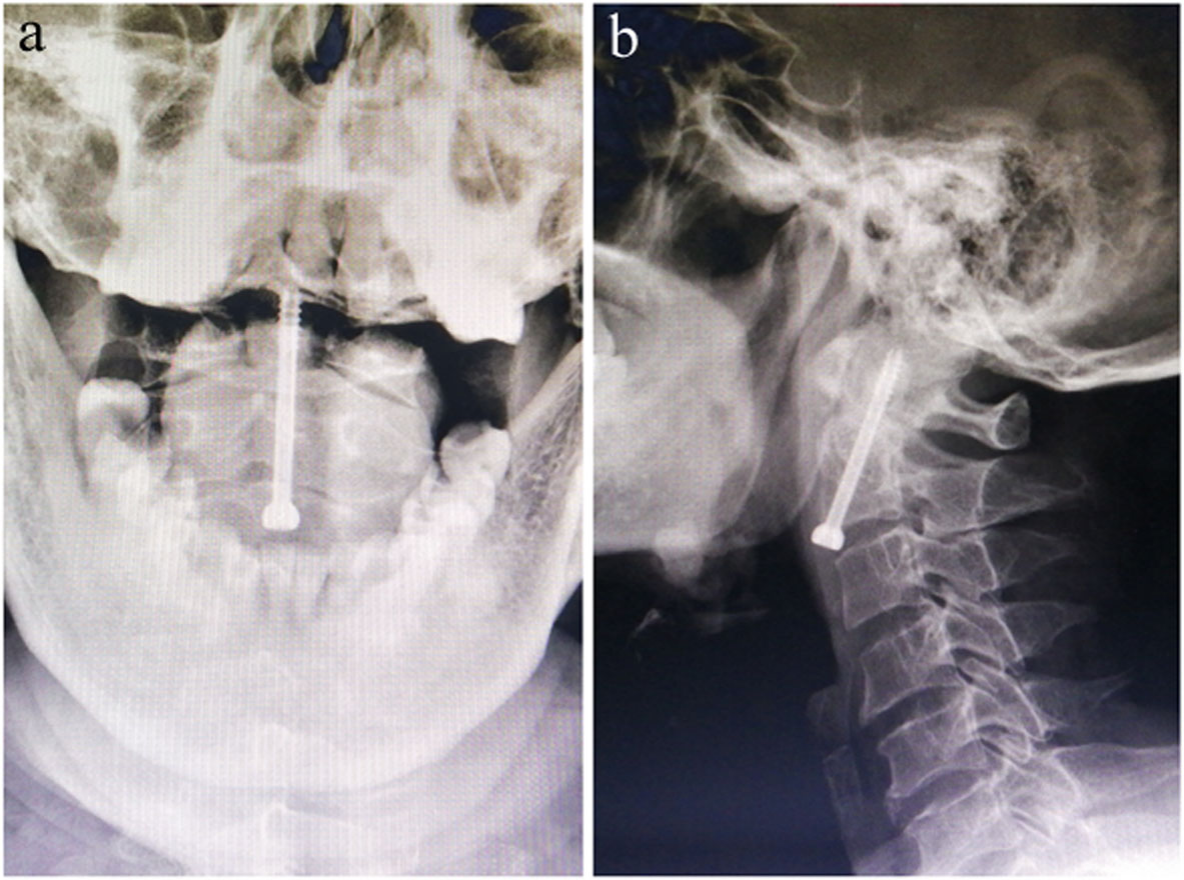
The anteroposterior (a) and lateral (b) radiograph showed screw fixation and union of the odontoid fracture 3 months after surgery. The fracture line was unclear with bridging bone passing
